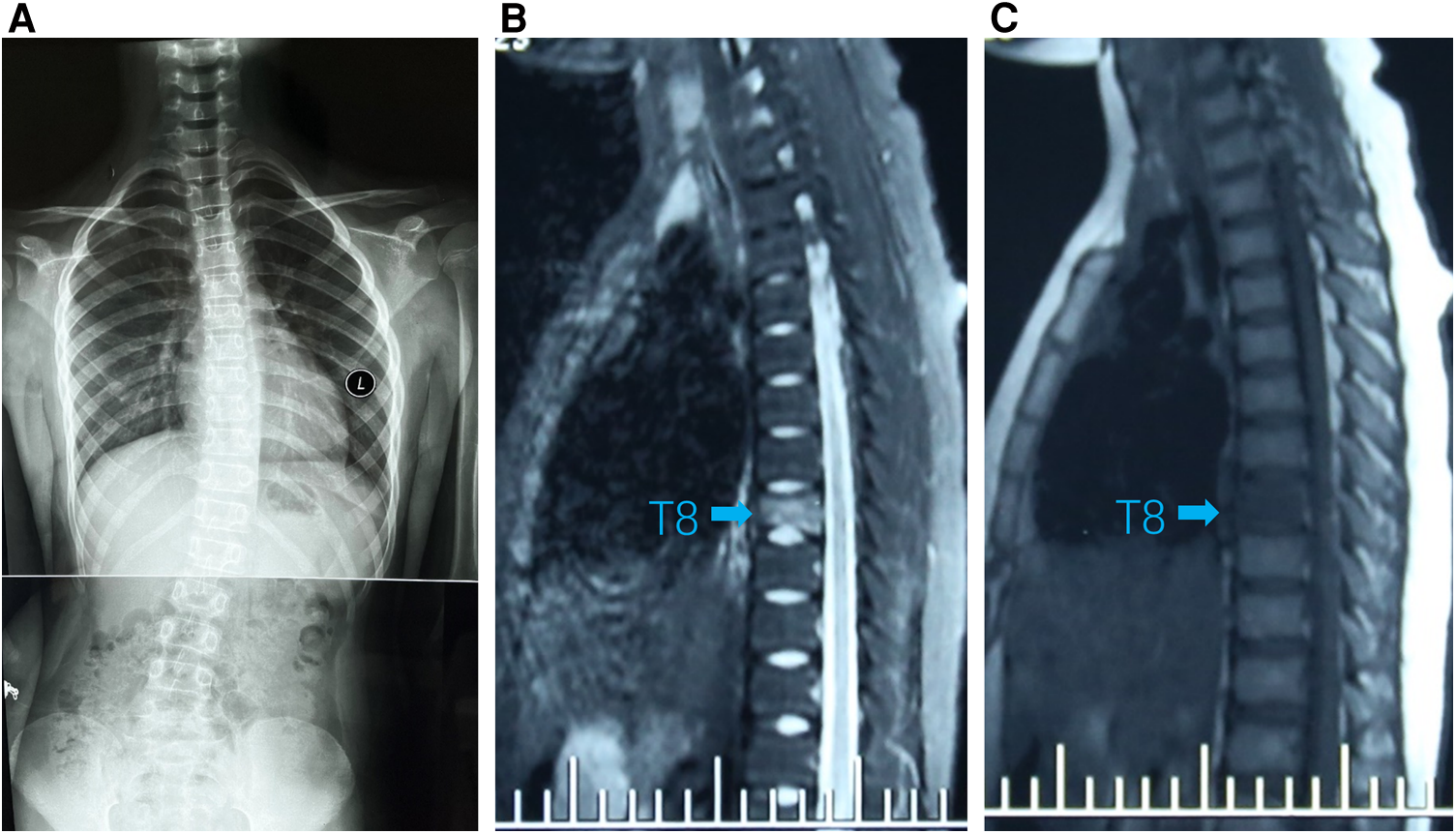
(A) Standing spinal x-rays showed right convex scoliosis. (B) T1-weighted MRI image showed that the T8 vertebra was flattened and local bone was destroyed (arrow). (C) T2-weighted MRI image showed bone marrow edema in T8 vertebra (arrow).
